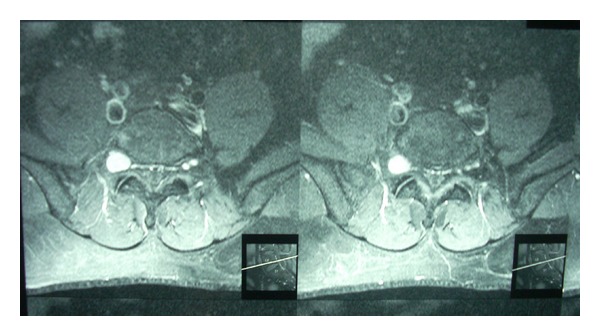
MRI T2W image (axial) shows hyperintense nerve tumour of the L5 nerve root in the L5-S1 neural foramina.
